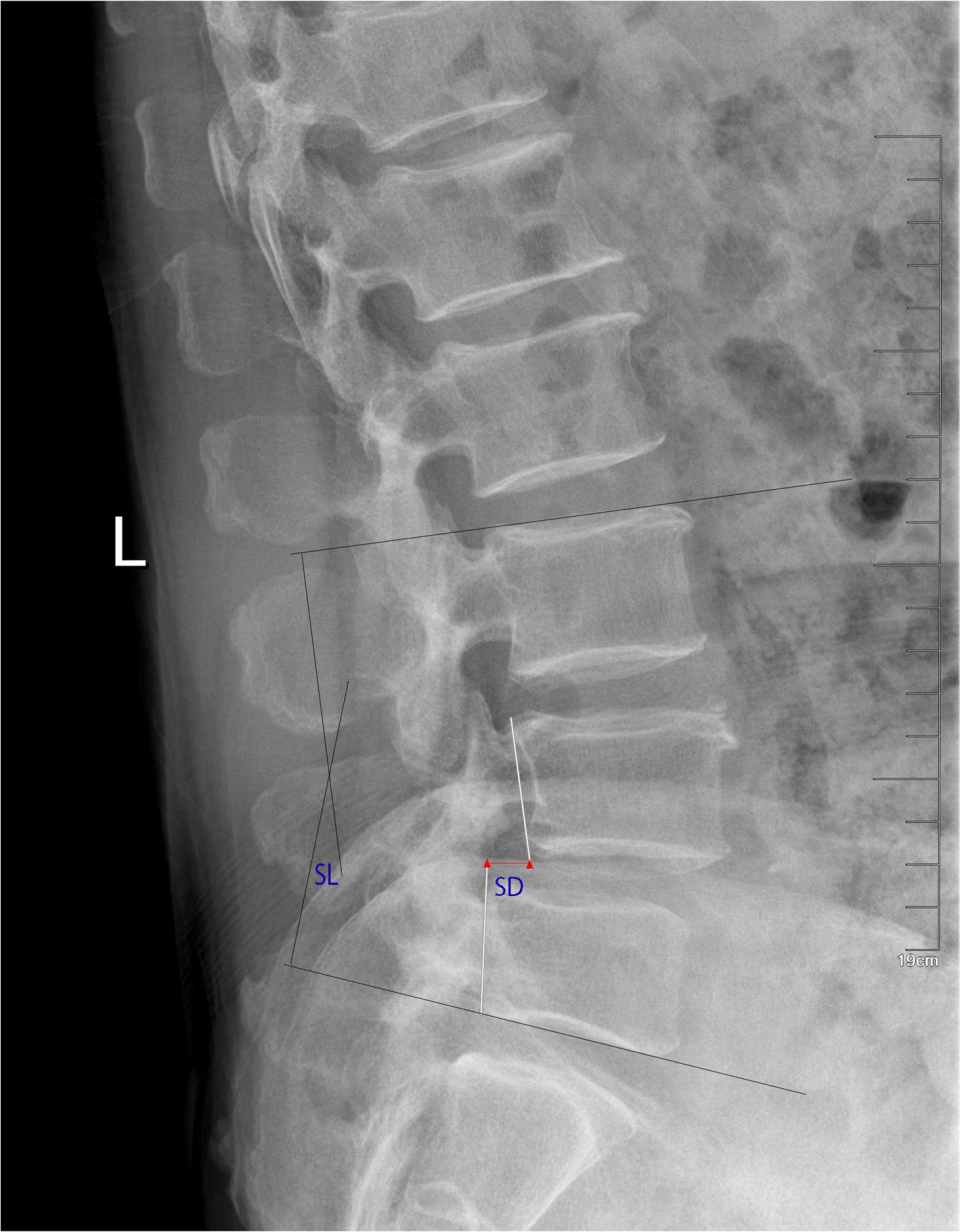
Plain lateral radiographs for measuring local deformity sparameters of spondylolisthesis. SD: Slip degree; SL: Segment lordosis
